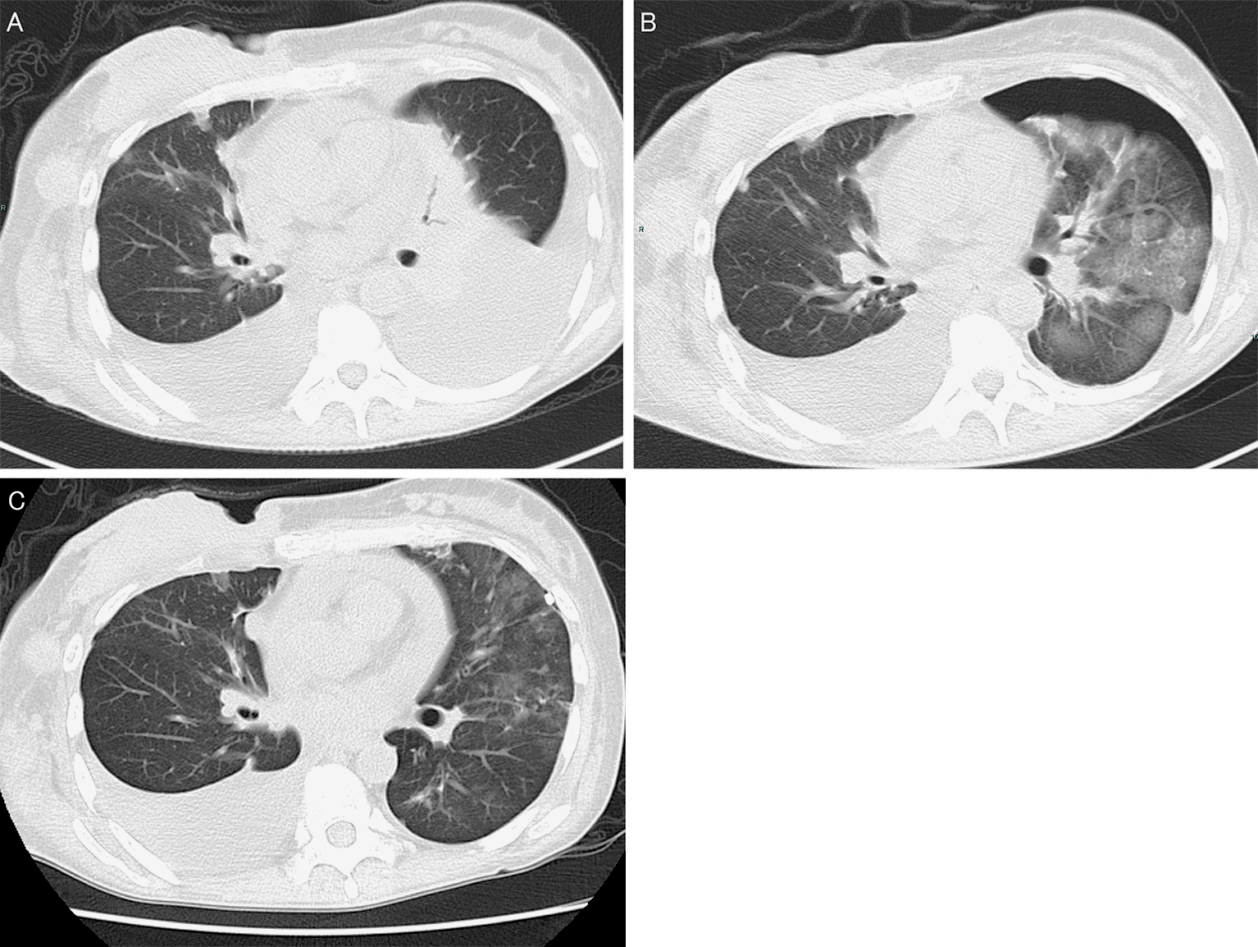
Computed tomography showing the onset of reexpansion pulmonary edema and improvement (A) Pre-drainage (B) Post-drainage (C) After 4 days.
